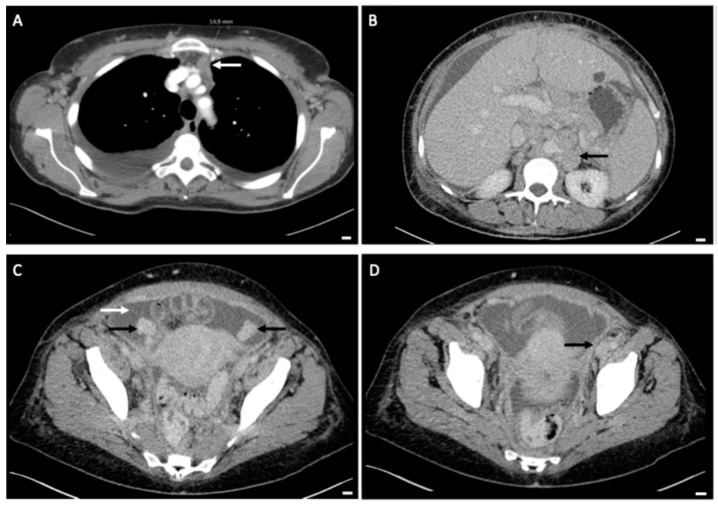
Preoperative CT-imaging of thorax and abdomen. (A): Up to 15 mm pathologically enlarged retrosternal lymph node (white arrow). (B): Bulky lymph nodes paraaortal left with single lymph nodes enlarged up to 2.8 cm (black arrow). (C): Solid, up to 3 cm enlarged ovaries on both sides (black arrows), ascites (white arrow). (D): Thickened peritoneum in the small pelvis, potentially indicative of peritoneal tumor metastases (black arrow). All white bars in the bottom right-hand corner indicate 1 cm.
